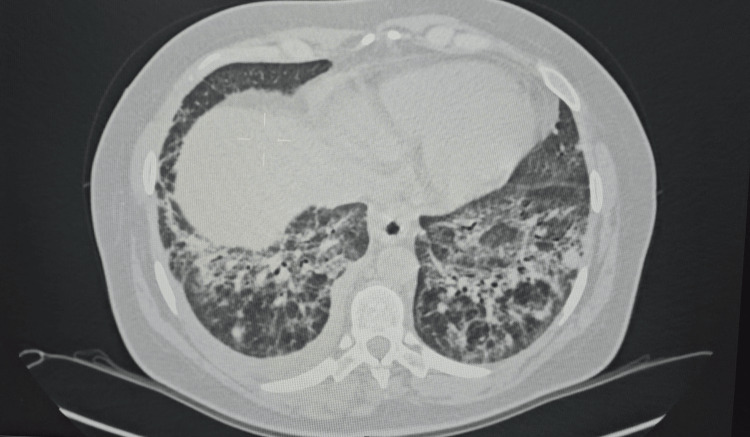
High-resolution computed tomography Parenchymal consolidation with ground-glass opacity diffusely involving the middle lung fields bilaterally and a 6 mm soft-tissue nodule located in segment 6 (S6) of the left lung
